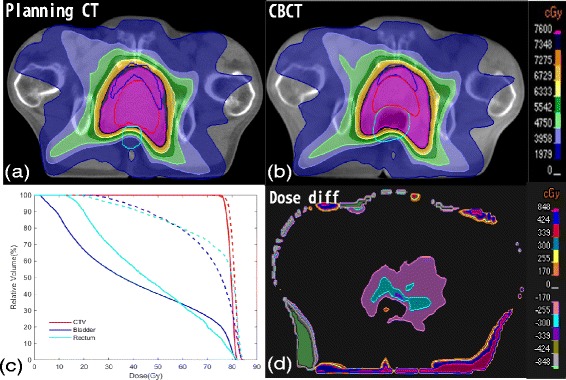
a–b represent dose distribution of the same transversal plane of the same patient in one of CBCT and CT respectively. c shows the DVH of the (a) (*solid line*) and (b) (*dash line*). d displays the dose difference between the (a) and (b)
